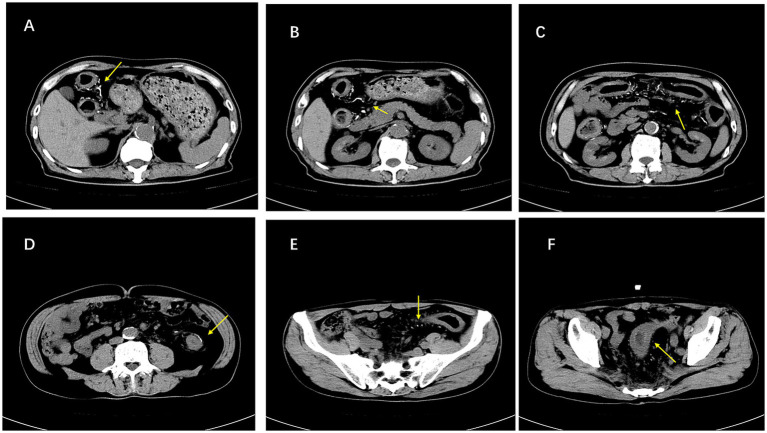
Abdominal CT images of idiopathic mesenteric phlebosclerosis (IMP). Non-contrast abdominal CT findings in IMP. (A–D) Axial images demonstrate circumferential thickening of the colonic wall with adjacent pericolic fat stranding, along with many thread-like hyperdense calcifications distributed along the pericolic and mesenteric veins (arrows). (E,F) Axial pelvic images show edematous thickening of the rectal wall with adjacent linear mesenteric/perirectal venous calcifications (arrows), consistent with IMP-related chronic ischemic change.
